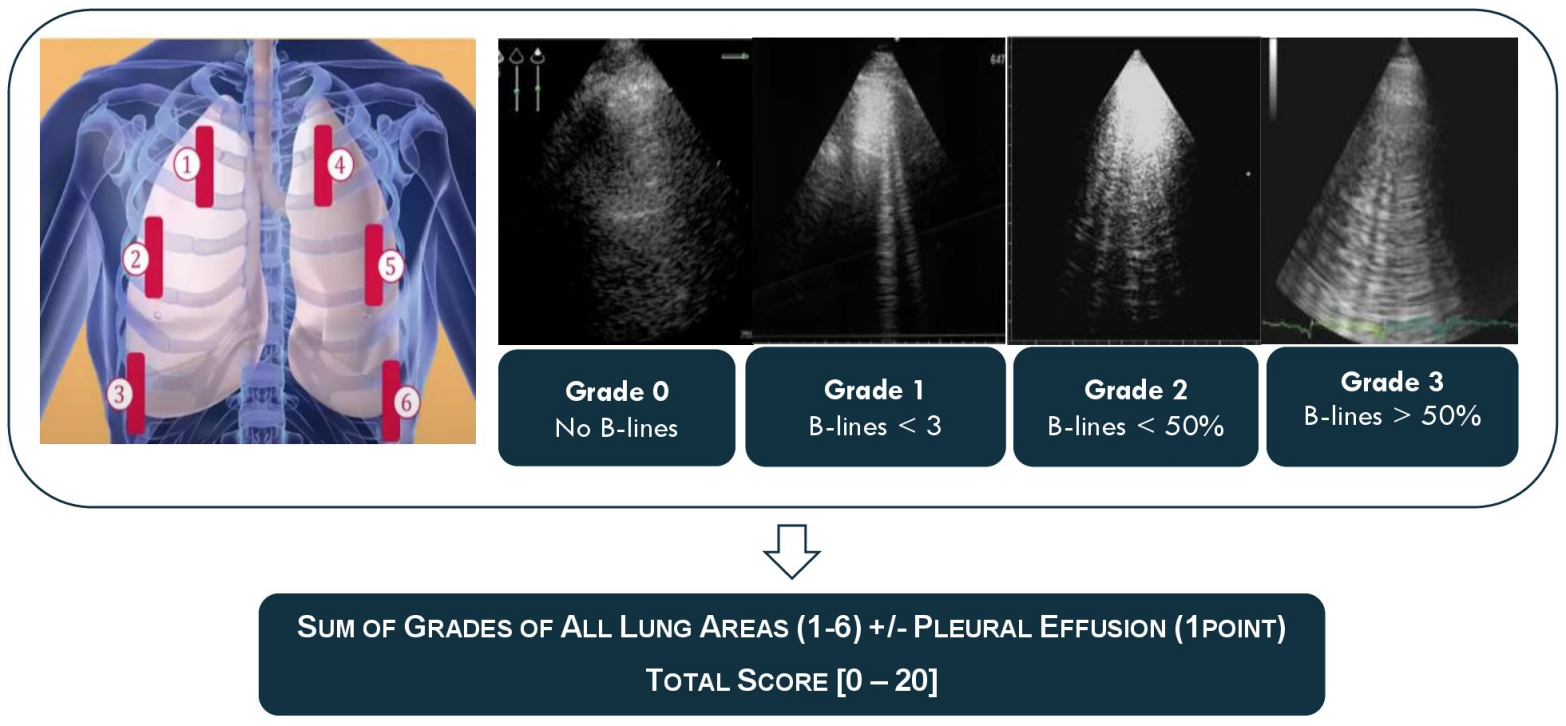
Modified Lung Ultrasound Score.
